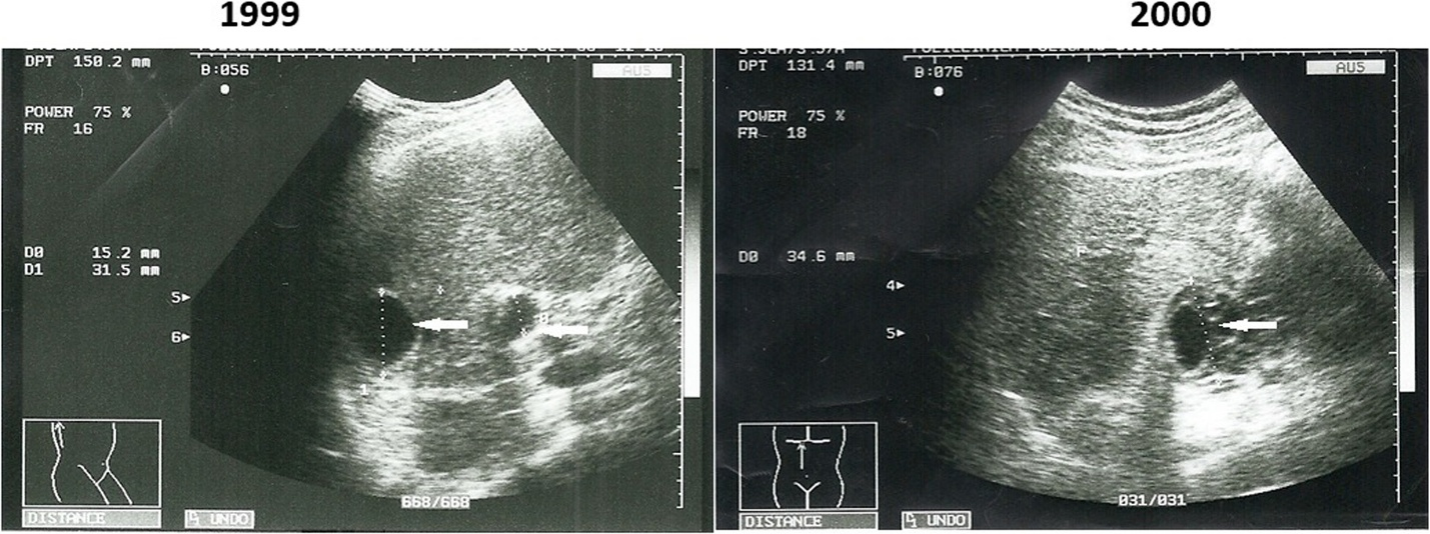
Ultrasound images after initial diagnostic.
